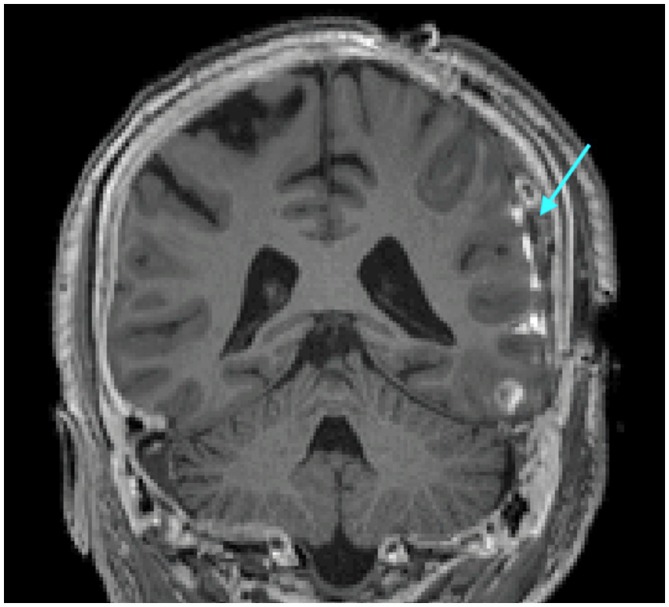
Coronal slice of the preimplant T1‐weighted magnetic resonance imaging coregistered with the postimplant computed tomography, demonstrating a visible implanted intracranial subdural electrode contact (arrow).
